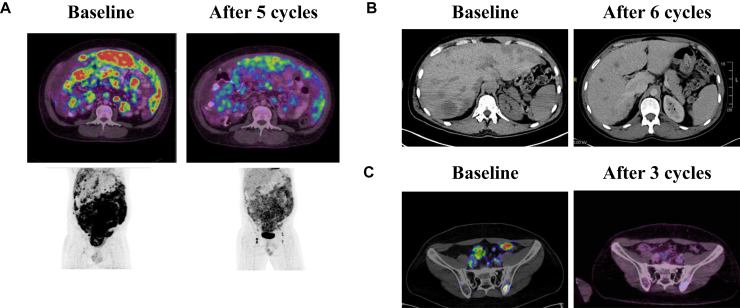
Radiological evaluation of response to treatment. (A) 18F-fluorodeoxyglucose-positron emission tomography (FDG-PET) in P1 at baseline and after five cycles of trastuzumab deruxtecan, displaying partial metabolic response on peritoneal sarcomatosis. (B) CT in P2 at baseline and after six cycles of trastuzumab deruxtecan, displaying partial response with both regression and disappearance of liver lesions. (C) FDG-PET in P3 at baseline after three cycles of trastuzumab deruxtecan, displaying partial major metabolic response with both regression of a bone lesion and disappearance of a peritoneal lesion. CT, computed tomography; P, patient.
